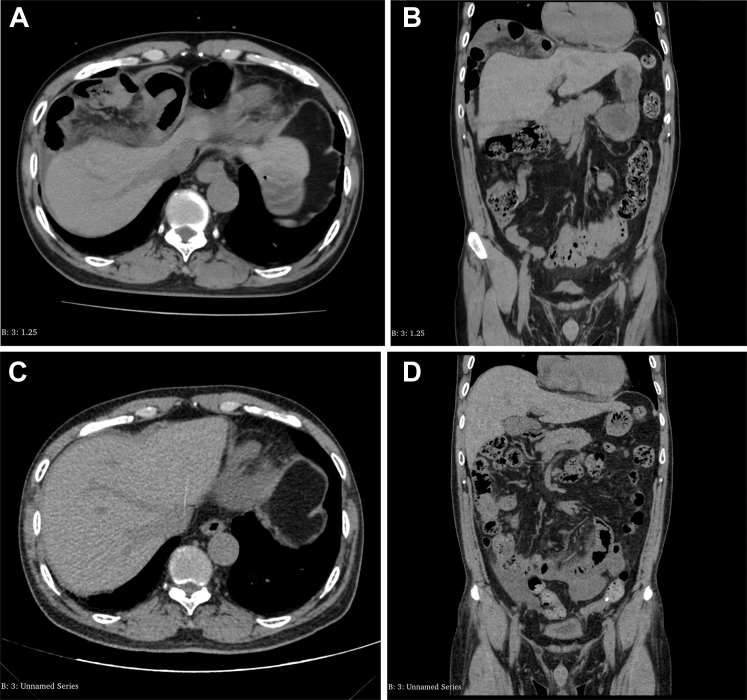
A, Axial view of the patient’s abdomen on computed tomography (CT) imaging. B, Coronal view of the patient’s abdomen on CT imaging. C, Axial view of the patient’s abdomen on CT imaging. D, Coronal view of the patient’s abdomen on CT imaging.
